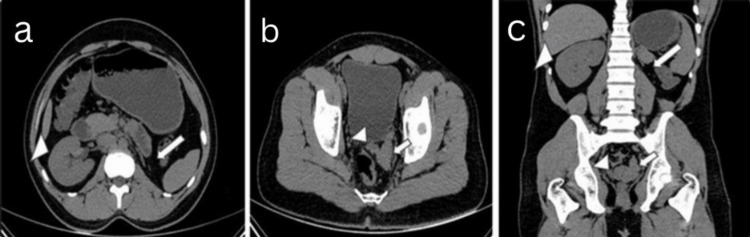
A 24-year-old male presented with a history of recurrent lower urinary tract infections associated with dysuria, frequency, perineal pain, and irritation following ejaculation over the past six months. Plain CT of the abdomen in (a,b) axial and (c) coronal views showed non-visualization (absence/agenesis) of the left kidney in the left lumbar region (large thick white arrow) and left seminal vesicle was enlarged with cystic changes (small thick white arrow), right kidney was normal (large white arrowhead), right seminal vesicle was normal (small white arrowhead). CT: computed tomography
